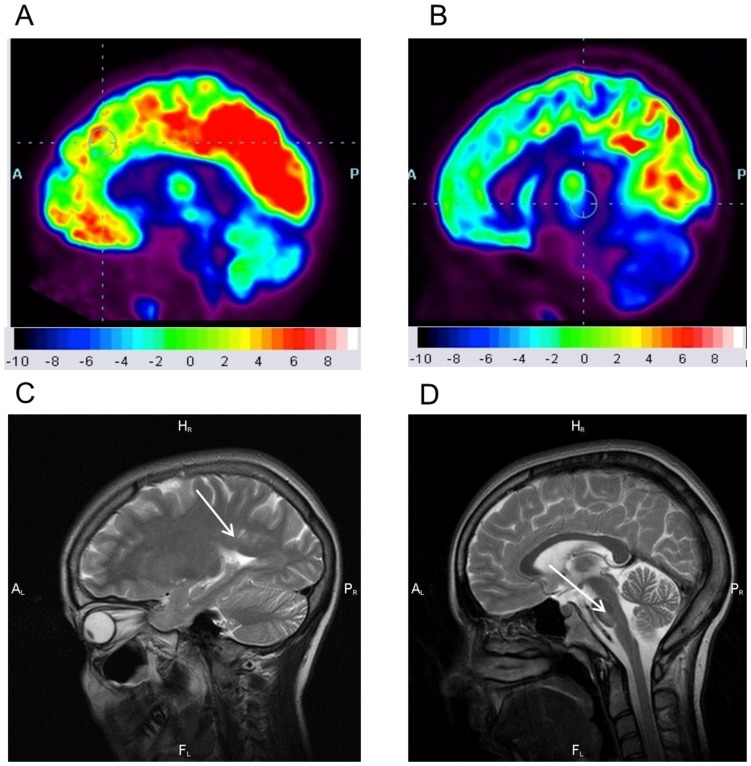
Differences in FDG uptake in PET-CT scans of the brain between a healthy child (a) and WFS6 patient (b) and small lesions in MRI scans in WFS6 patient in the paraventricular white matter (c) and brain stem (d).
